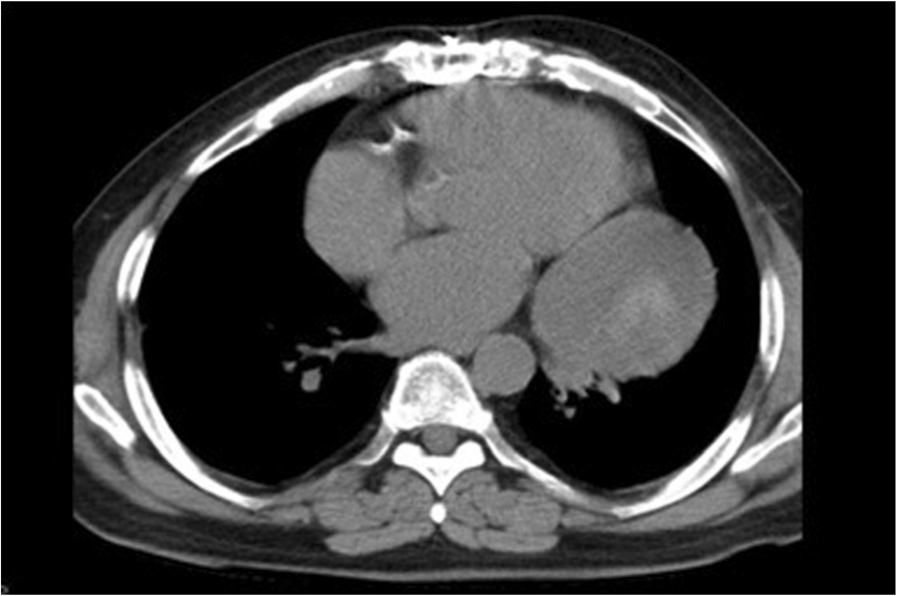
The Chest CT demonstrates a mass with smooth edges and heterogeneous density, next to the heart and partially intrapericardial
